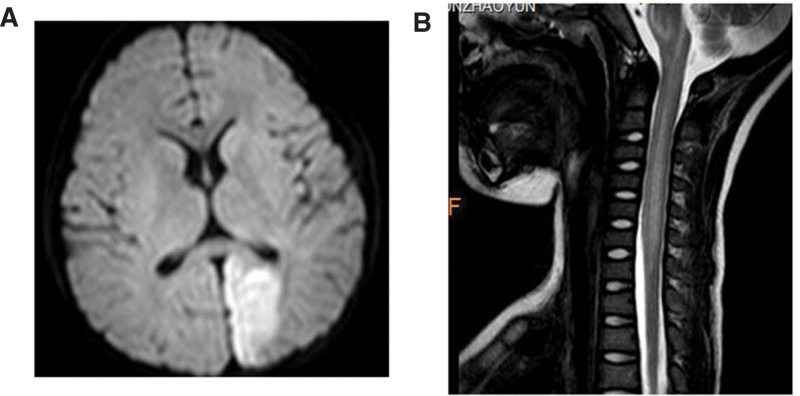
MRI imaging of the patient. (A) MRI of the brain showed patchy iso-intense T1 and iso-intense T2 signals in the left parietal occipital lobe. (B) MRI of the cervical spine showed longer T1 and T2 signals in the medulla oblongata to C7 cervical vertebrae with the presence of local edema. MRI = magnetic resonance imaging.
